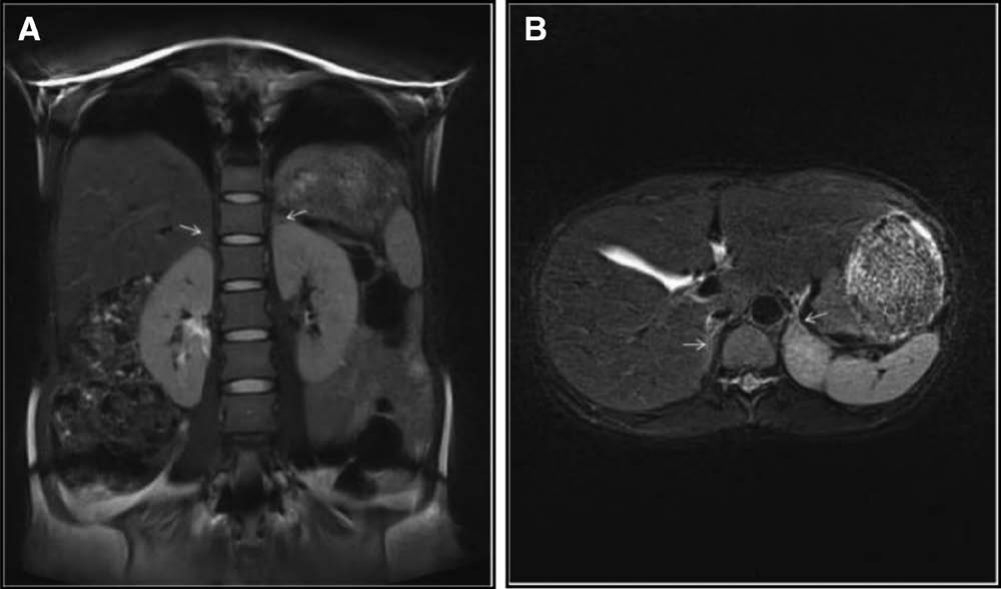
Antenatal MRI scan in the adrenal gland. (A) In the fat-suppressed coronal position image, the junction region between the 2 glands was obvious (as noted by the white arrow), without the presence of abnormal shape or signals. (B) In the fat-suppressed and T1W1 position image, the boundary of the 2 glands was clear, with the lateral limb in the right gland being smooth and linear and that in the left gland being triangular. No abnormal shapes or signals were observed (as noted by the white arrow).
